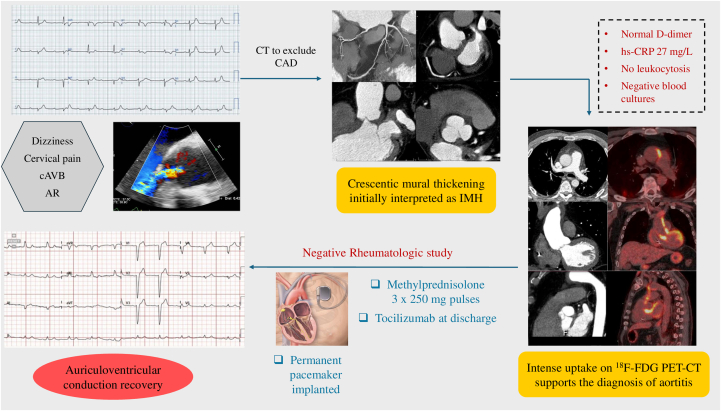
Clinical Timeline of Primary Aortitis Presenting With cAVB AR = aortic regurgitation; cAVB = complete auriculoventricular block; CAD = coronary artery disease; hs-CRP = high-sensitive C-reactive protein; CT = computed tomography; IMH = intramural hematoma; ^18^F-FDG PET-CT = fluorine-18 fluorodeoxyglucose positron emission tomography-computed tomography.
